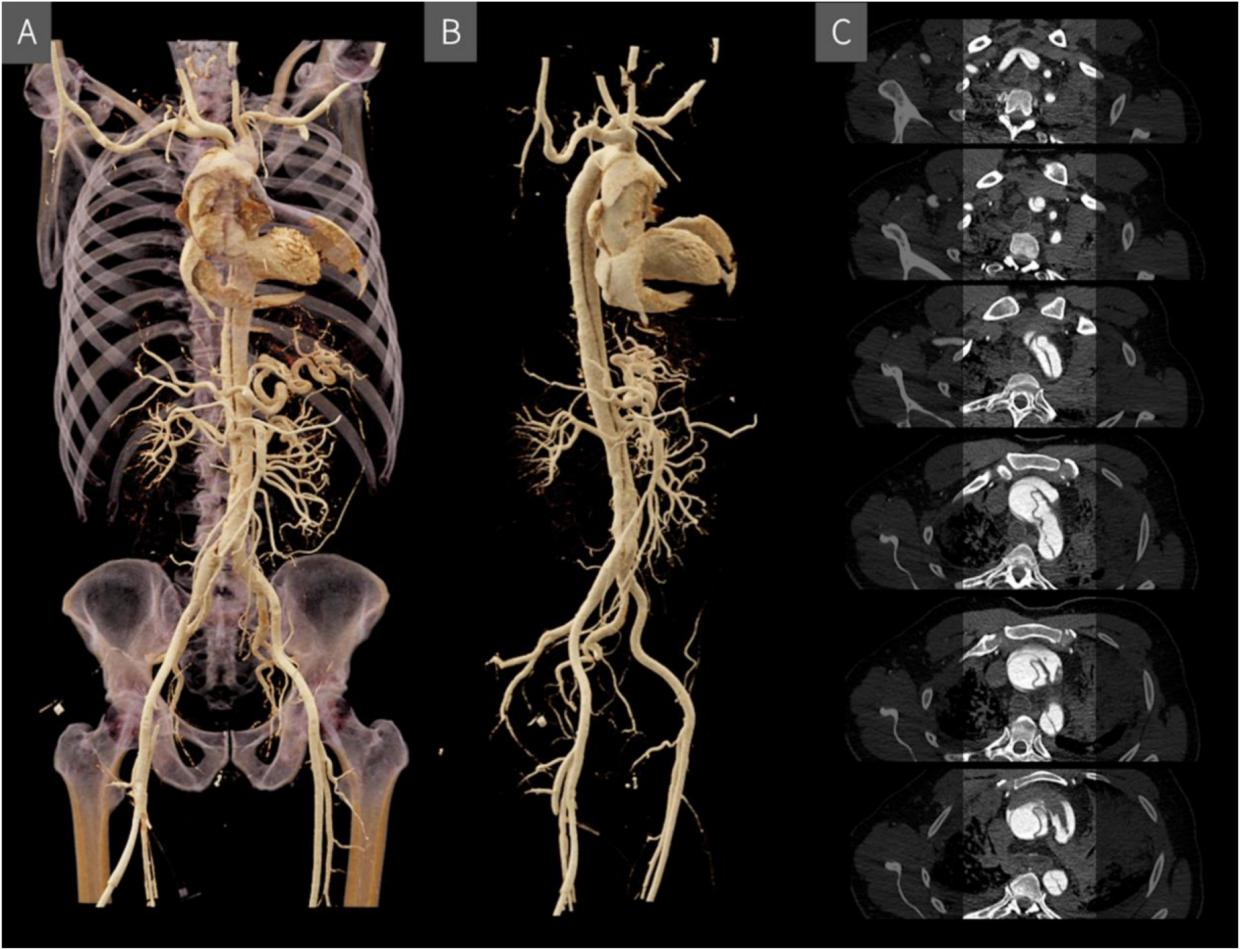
Post-mortem CT angiography visualized in 3D using cinematic rendering (A and B) and in individual cross-sectional slices (C) the rupture site is located above the valvular plane, extending ventrally with hemorrhage into the pericardial and mediastinal spaces. A Stanford type A aortic dissection originates at the level of the valvular plane/aortic root and continues supra-aortically, involving the brachiocephalic trunk and extending into the right subclavian artery, with a short segment also affecting the left subclavian artery.
